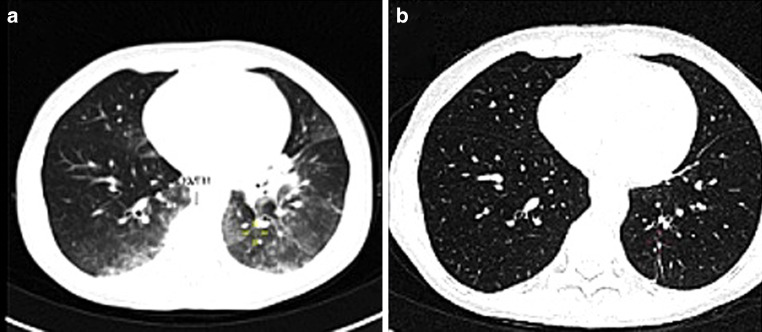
a Thoracic CT on January 23, 2025, showing immunotherapy-induced pneumonitis. b Follow-up CT on January 31, 2025, after corticosteroid therapy, showing resolution of pulmonary infiltrates
